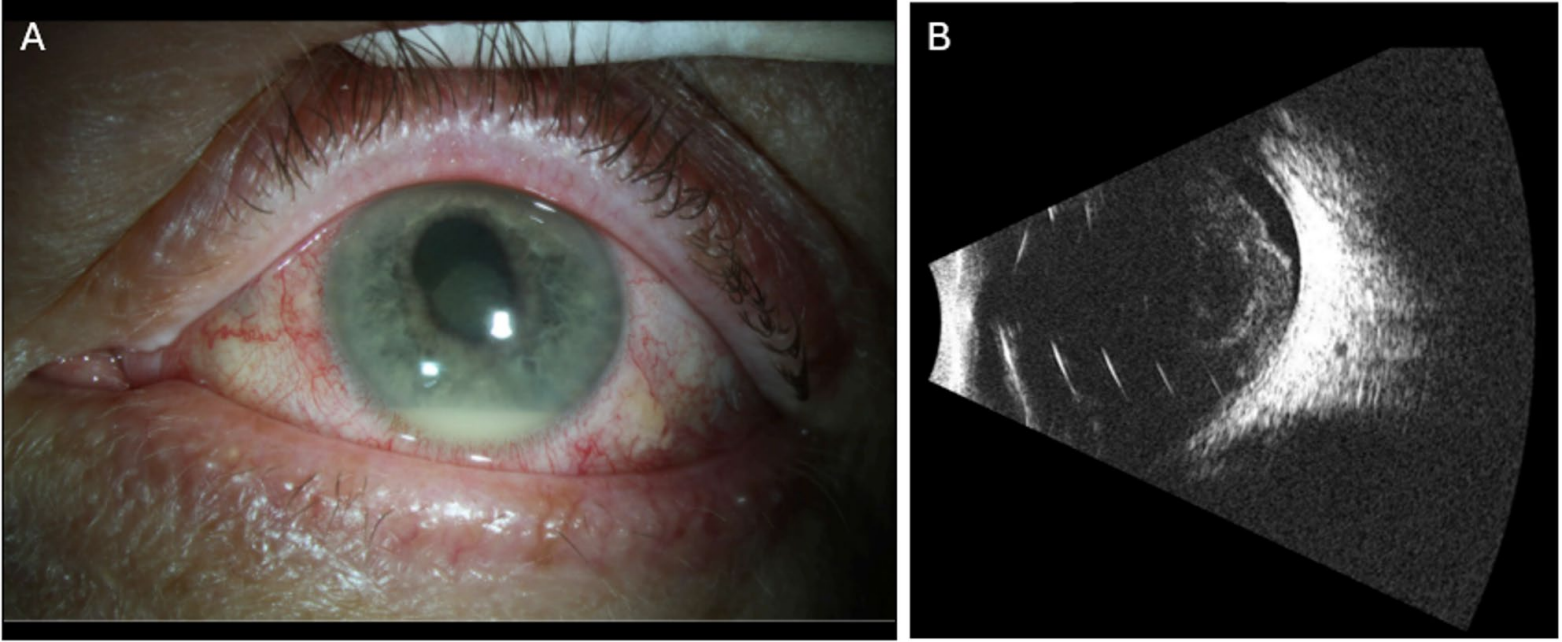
Patient 6: A 64-year-old male presented 5 days after receiving intravitreal bevacizumab in the left eye. Visual acuity was hand motions. (A) Examination demonstrated diffuse conjunctival injection, corneal infiltrate, and a 2 mm hypopyon. (B) B-scan ultrasound demonstrated dense vitreous debris and an incomplete posterior vitreous detachment. The patient underwent vitreous tap and injection with vancomycin, ceftazidime and dexamethasone and eventual pars plana vitrectomy with repeat injection of intravitreal vancomycin, ceftazidime and dexamethasone. Best-corrected visual acuity at last follow-up 6 days after initial presentation was hand motions.
